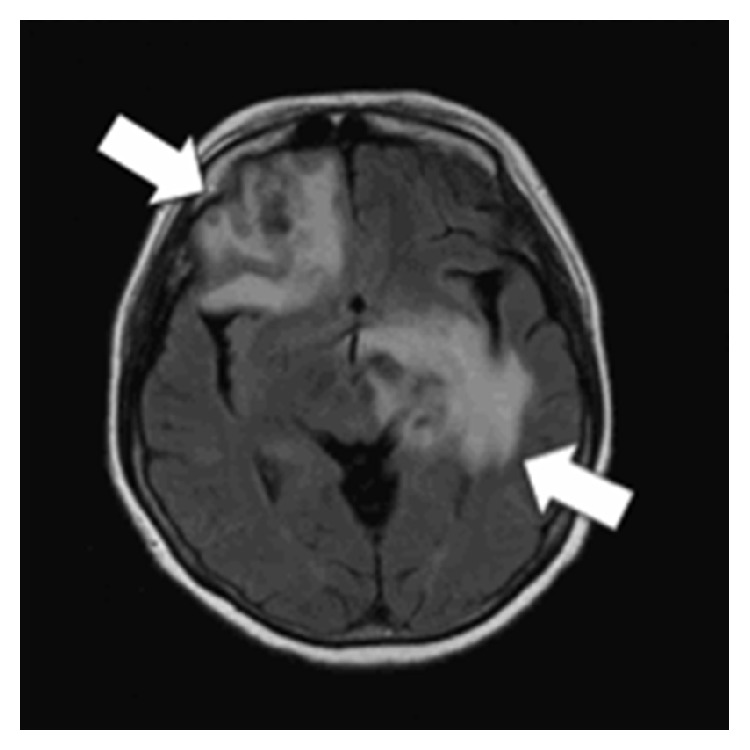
Fluid attenuation inversion recovery- (FLAIR-) weighted MR imaging of brain with contrast showed multiple infiltrative lesions (arrows) in the left basal ganglion, left thalamus, and right frontal periventricular white matter.
